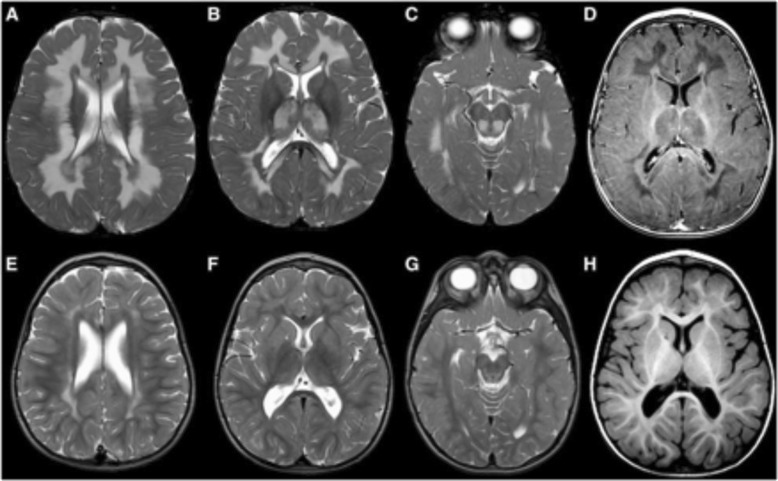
Brain T2-weighted MRI representative of mild LTBL. Axial T_2_- (a–c and e–g) and T_1_-weighted images (d and h) in (EARS2) patient 6 with a mild disease course at 11 months (a–d) and 3 years (e–h). Note the extensive T_2_-hyperintense and T_1_-hypointense signal of the deep cerebral white matter with sparing of a periventricular rim (a, b, and d). There are also signal abnormalities in the thalami (b) and dorsal part of the midbrain (c). Note the impressive improvement 2 years later (e–h). Reprinted with permission [45]
